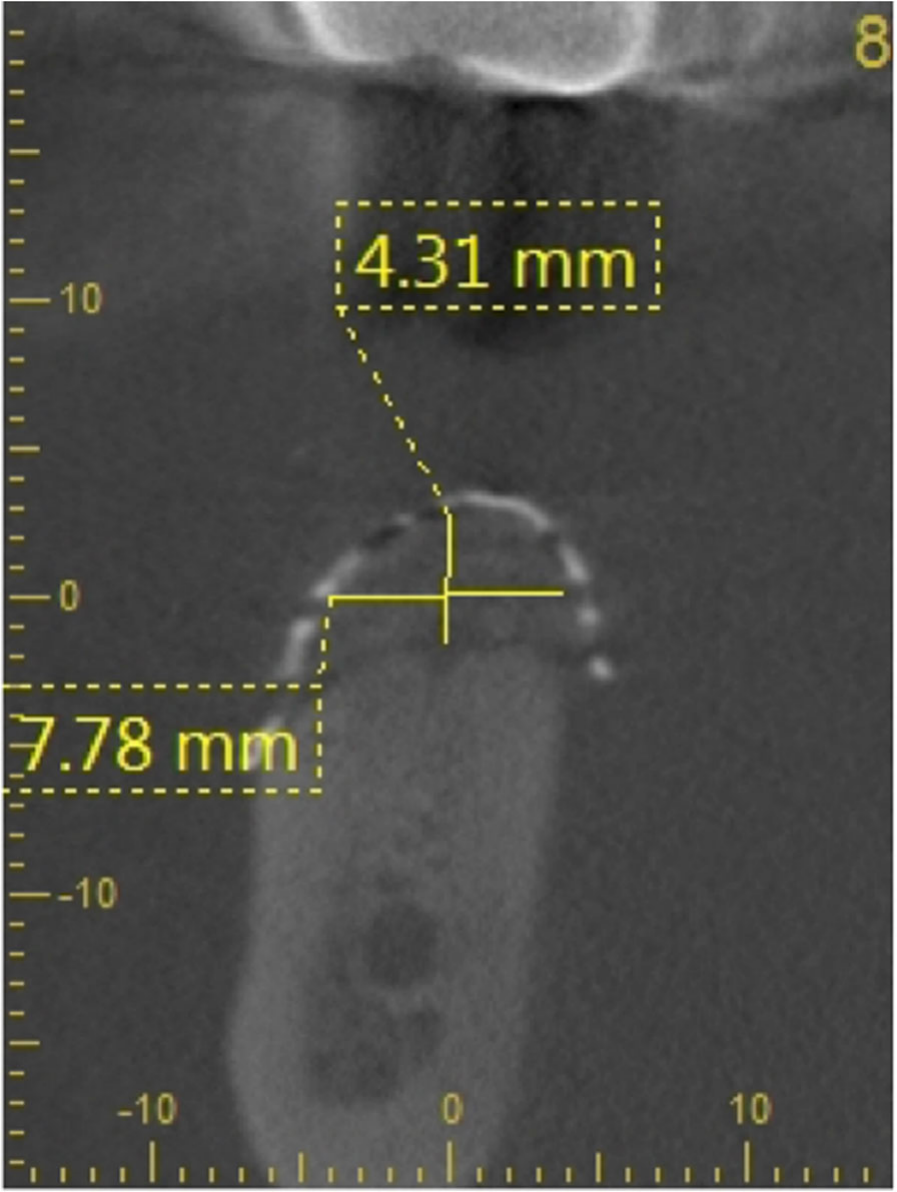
Representative picture of analysis of vertical and horizontal bone augmentation on CBCT scan
